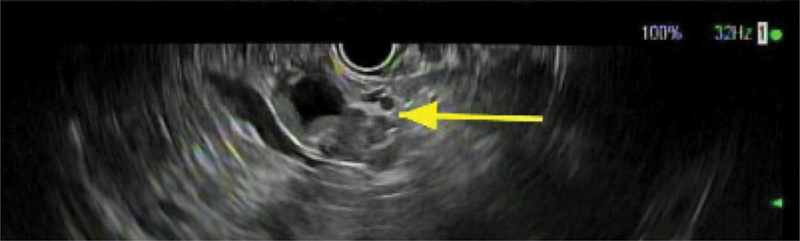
Atypical cystic pancreatic tail lesion on EUS with solid components. EUS, endoscopic ultrasound.
